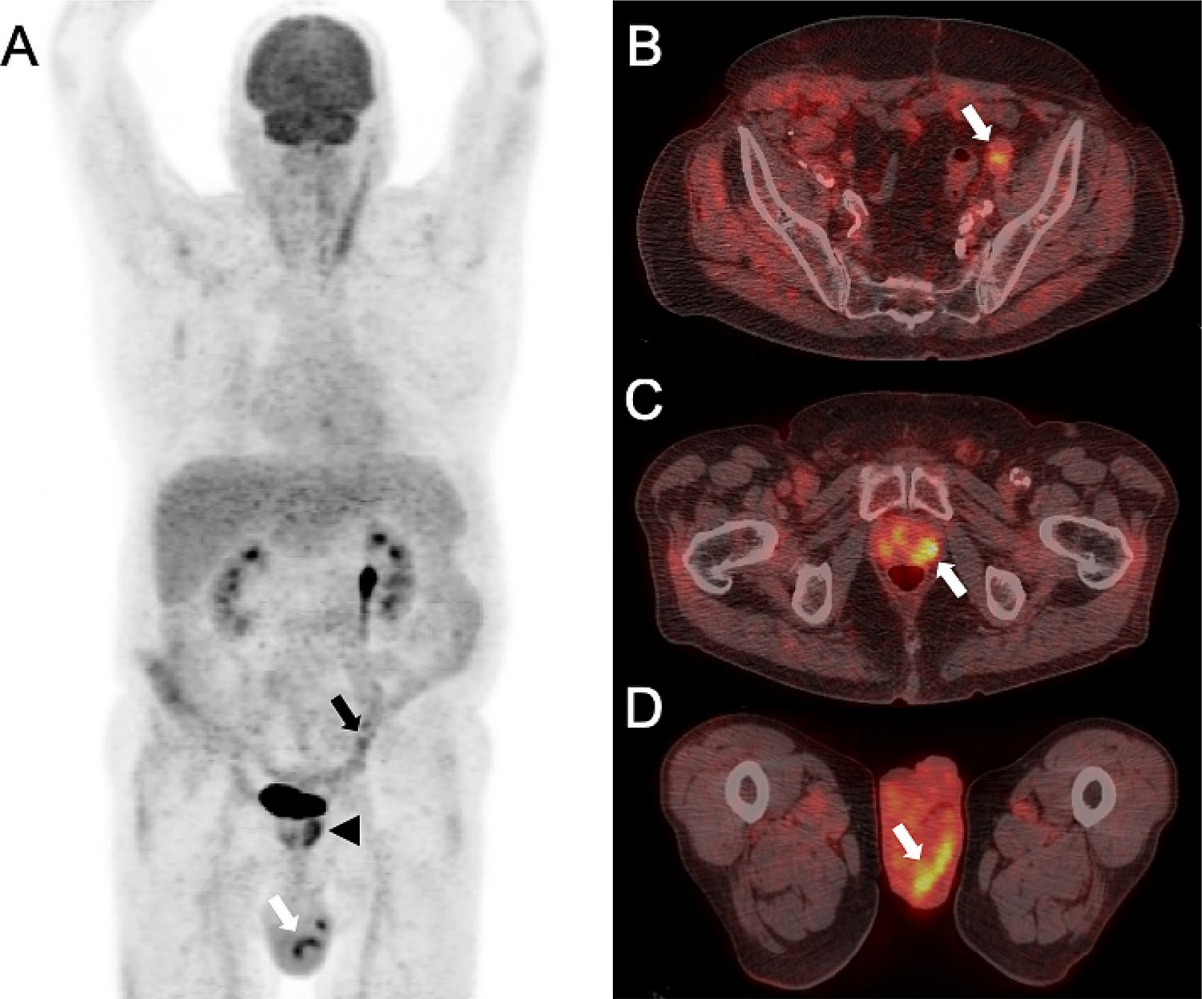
A 61-year old male patient was referred to PET/CT one year and four months after the initial diagnosis of a vascular endograft infection due to *Streptococcus dysgalactiae*. The patient was under antimicrobial therapy, but had acute fever and the reason for referral was the search for new infectious foci. PET/CT [maximum intensity reconstructions of PET (A) and fused PET/CT images (B–D)] showed faint residual focal FDG uptake along the extraanatomical aorto bi-iliac reconstruction (Dacron silver Intergard graft) (black arrow in A, white arrow in B), suggestive for persistent infection. Unknown and relevant incidental findings with impact on patient management were detected by PET/CT with intense focal FDG uptake in the prostate gland (black arrow head in A, white arrow in C), and in the epididymis (white arrows in A and D). Subsequent further work-up confirmed prostatitis with *Enterobacter cloacae* and antibiotic treatment was escalated accordingly.
